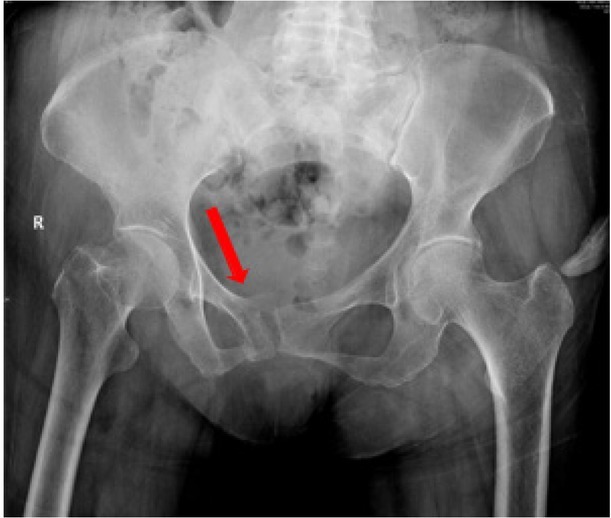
Pelvic X-ray showed mild displacement symphysis pubis fracture without dislocation of the joint.
